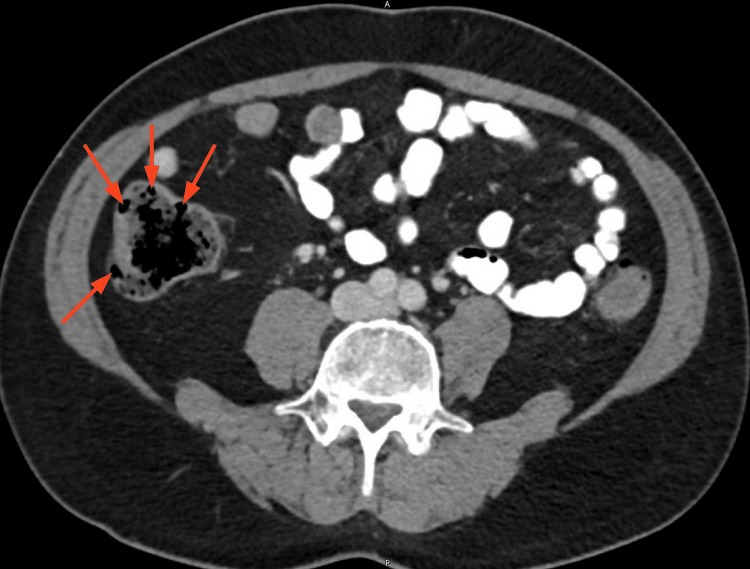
Axial CT scan of the abdomen showing cecal diverticulosis CT image demonstrating multiple cecal diverticula (red arrows), seen as air-containing outpouchings within the wall of the cecum.
